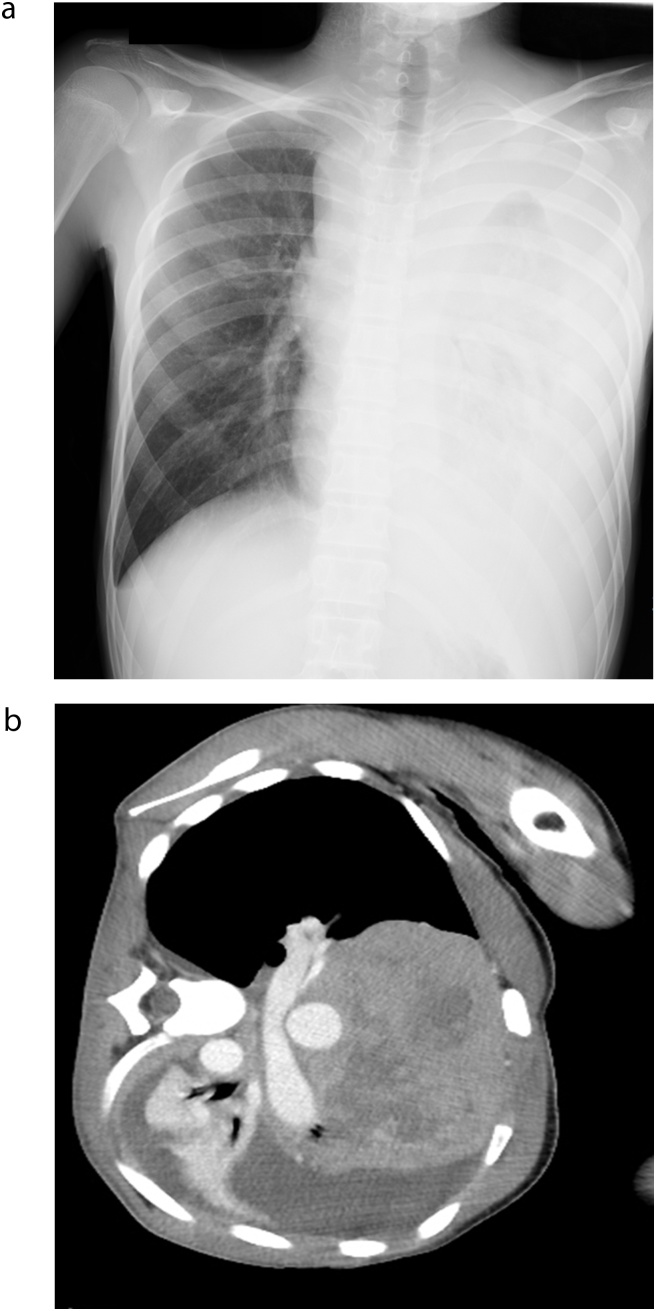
a. Chest X-ray showing an enlarged mediastinal shadow, left atelectasis, and pleural effusion. b. Chest computed tomography showing a large anterior mediastinal tumor, tracheal and cardiovascular compression, left pleural effusion, and atelectasis. The image is rotated because the patient’s respiratory distress was only relieved by placement in the left lateral decubitus position.
